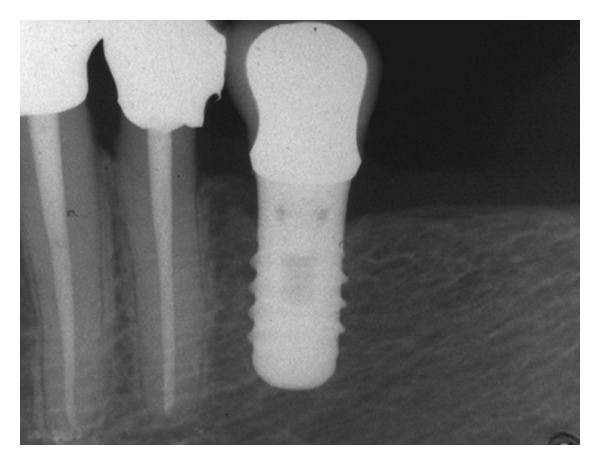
Single-tooth fixed prosthesis supported by a short implant (4.1 × 8 mm): 12 years after loading. Periapical radiograph.
